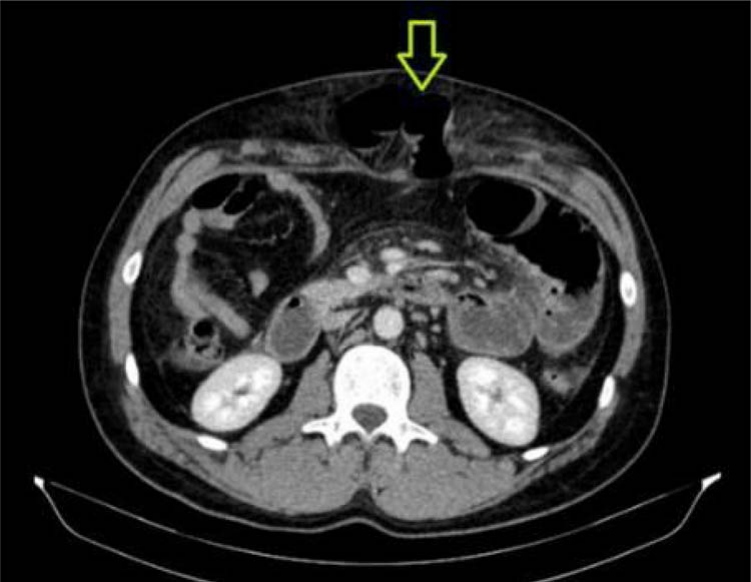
CT abdomen showing abdominal wall hernia containing a loop of small intestine (green arrow).
